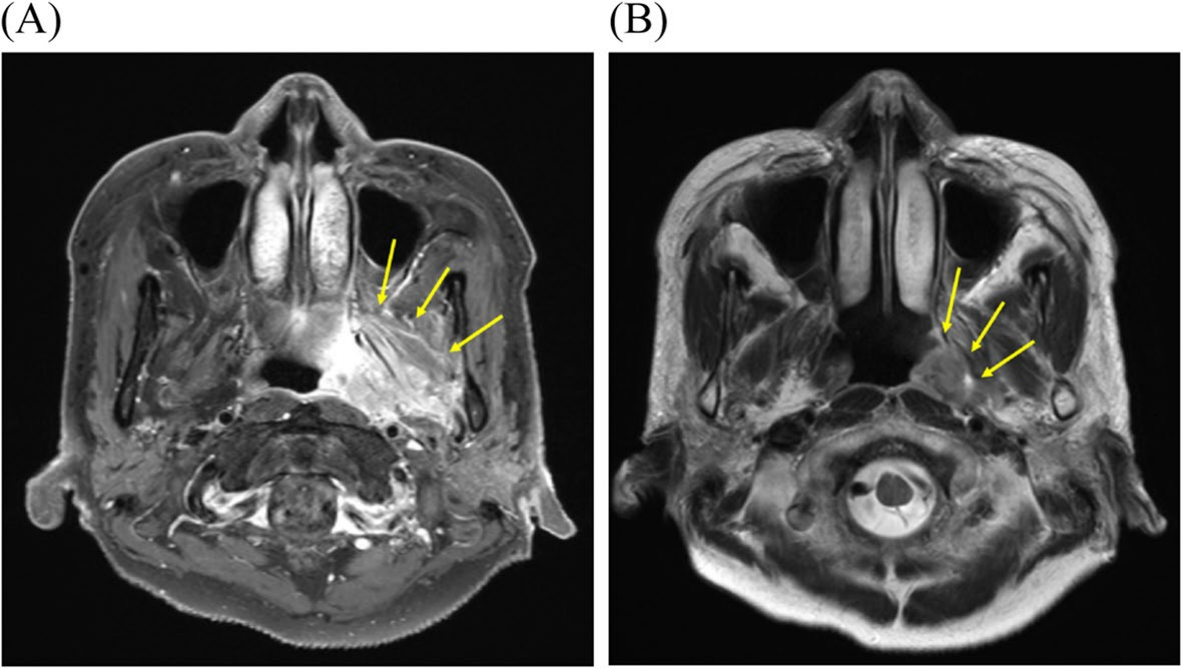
MRI images a month after the first visit (case 3). Increased signal reaction (yellow arrows) is observed in the left pterygoid and parapharyngeal area. A Triggered T1 image. B T2 image
